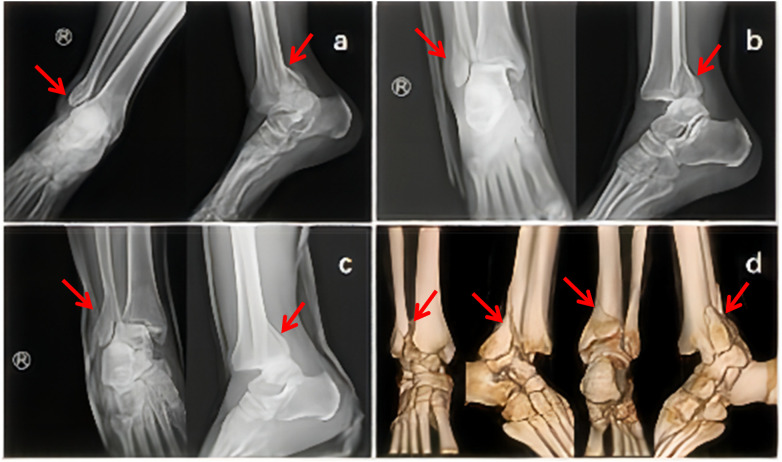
(a) X-ray showed fracture and dislocation of ankle joint at the time of treatment; (b) x-ray after the first reduction, fracture-dislocation was not corrected; (c) x-ray after the second reduction, fracture-dislocation was still not remedied; (d) the second review of CT with three-dimensional reconstruction was diagnosed as Bosworth fracture-dislocation.
